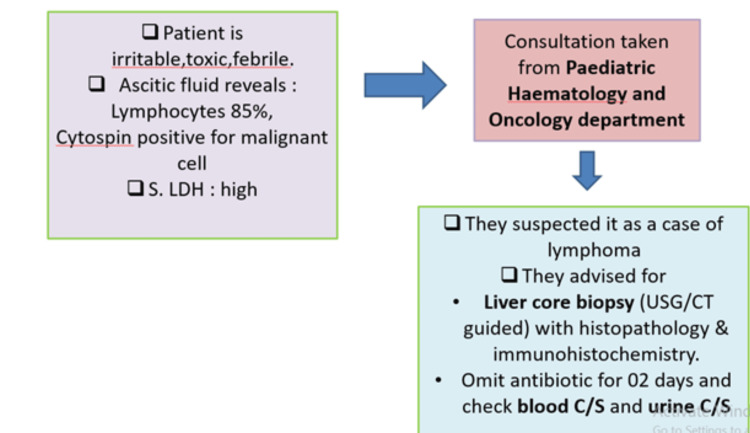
The treatment course for our patient on day six of hospitalization S. LDH: Serum lactate dehydrogenase; USG: Ultrasonogram and CT: Computed Tomography, Blood C/S: Blood culture, Urine C/s: Urine culture
